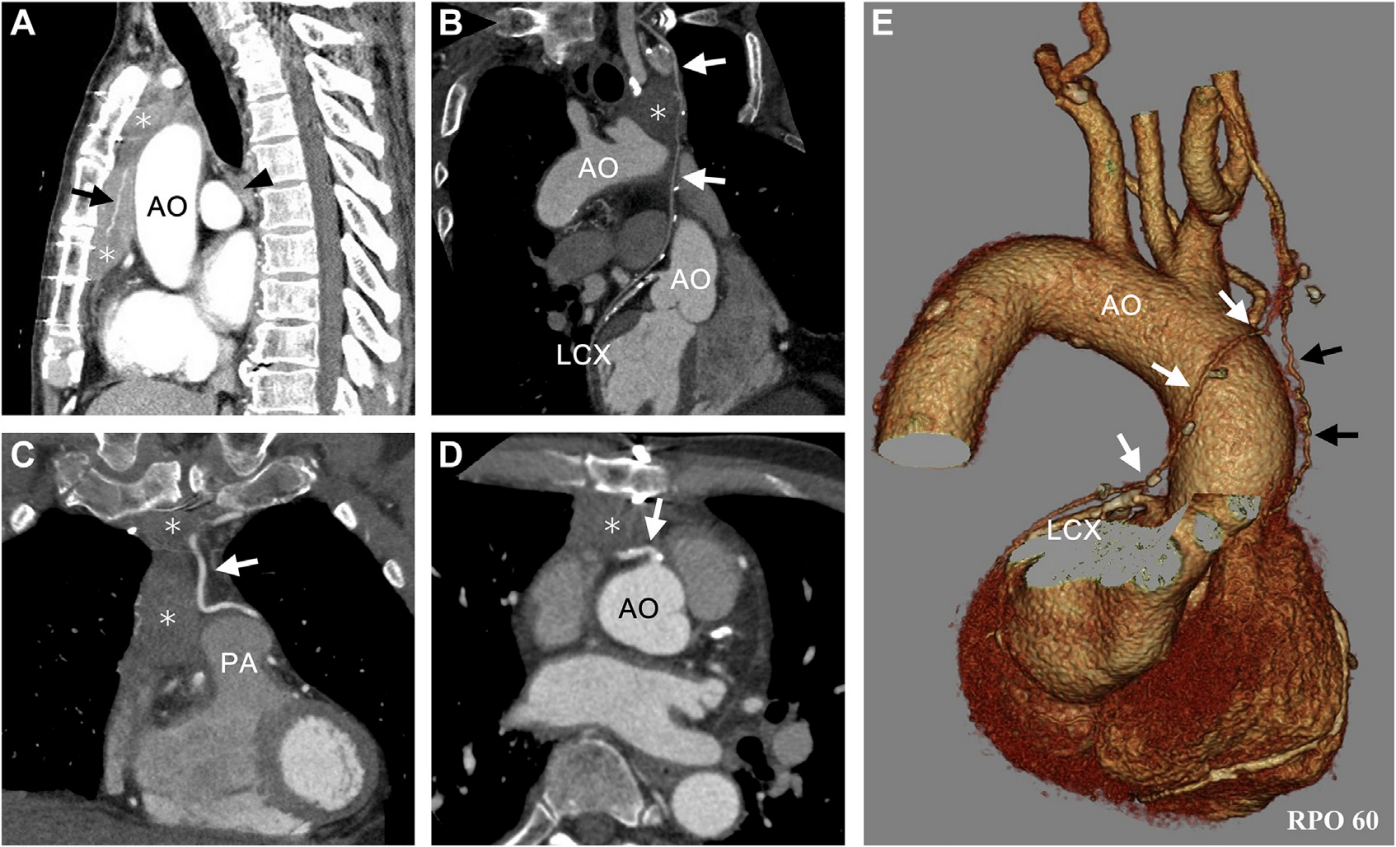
Contrast-Enhanced CT Images (A) A complex infiltrating anterior mediastinal mass (asterisks) behind the sternum. The smaller upper part and the larger lower part, wrapping around a large feeding vessel (arrow). An enlarged subcarinal lymph node suggesting lymph node metastasis (arrowhead) was observed. (B) Proximal right internal thoracic artery graft (white arrows) infiltrated by the tumor upper part (asterisk). (C) The tumor (asterisks) medially invaded the left internal thoracic artery graft (white arrow). (D) The tumor (asterisk) infiltrated the proximal right coronary artery (white arrow) and pericardium. (E) Three-dimensional CT reconstruction image showed a large feeding artery (black arrows) from the RITA (white arrows). AO = aorta; CT = computed tomography; LCX = left circumflex artery; PA = pulmonary artery.
